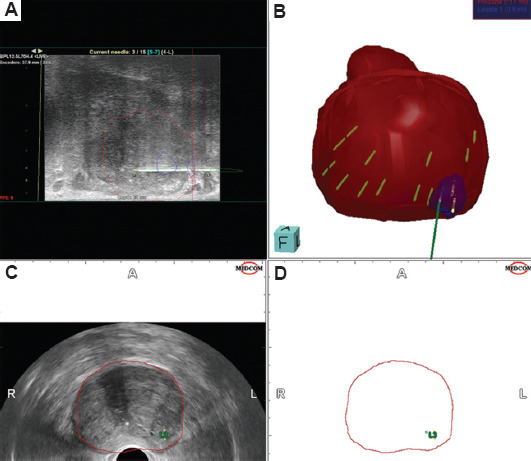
The process of magnetic resonance imaging/ultrasound (MRI/US) fusion-guided biopsy, combining targeted and systematic biopsies. (A) Targeted prostate biopsy of the suspected lesion obtained by needle under MRI/US fusion. (B) Three-dimensional model of the prostate (red), the suspected lesion (blue), and both systematic and targeted biopsies obtained. (C and D) Planning of the prostate borders and identification of the location of the suspected lesion. Red-marked area: prostate; blue-marked area: lesion; A: Anterior, L: Left; P: Posterior; R: Right.
